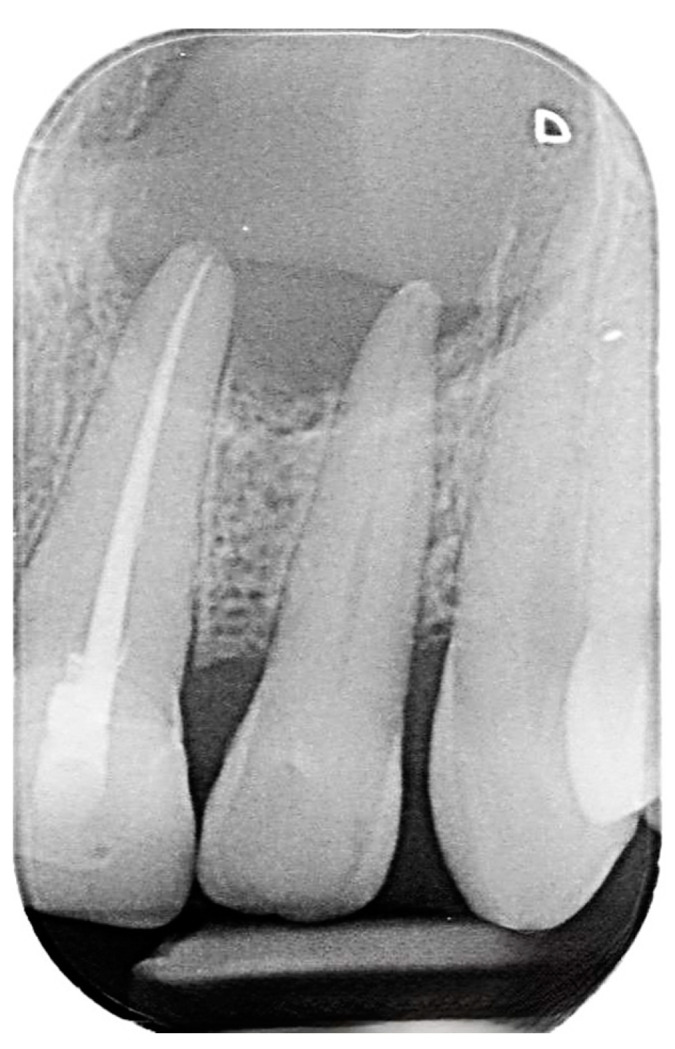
Pre-operative intraoral periapical radiograph of the upper left central and lateral incisors.
